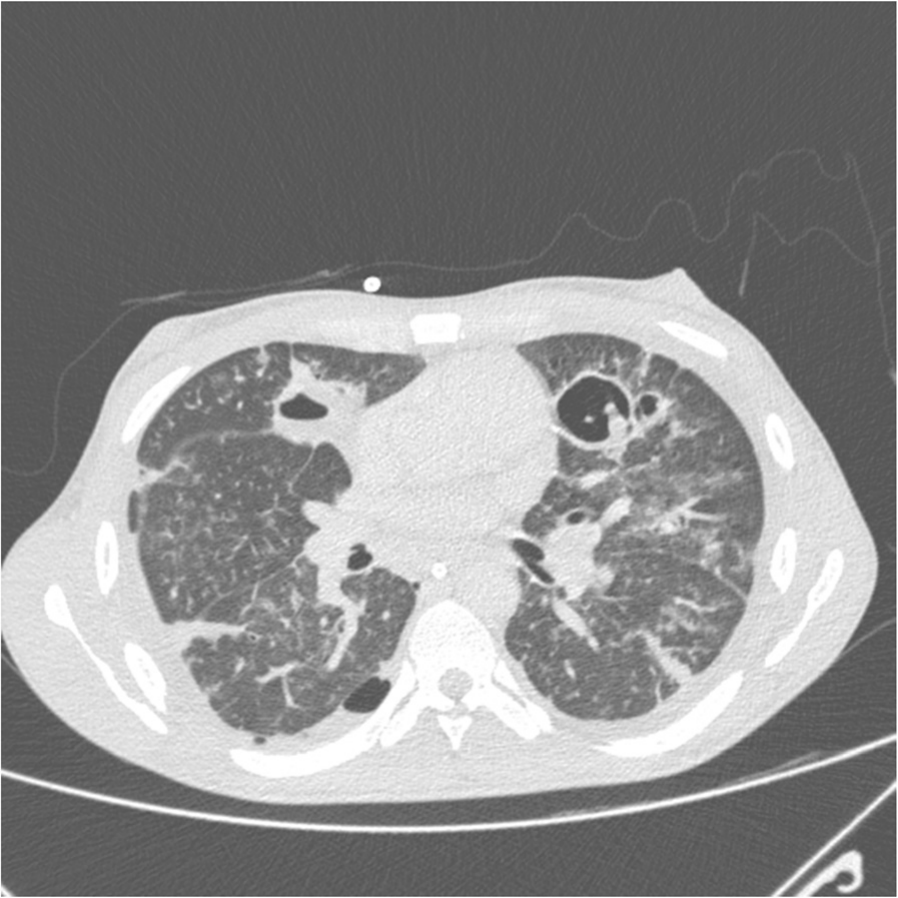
Middle third of the chest in computed tomography scan performed on the 11th day of hospitalization showed foci of pulmonary consolidation and cavities with thickened walls and fluid levels
